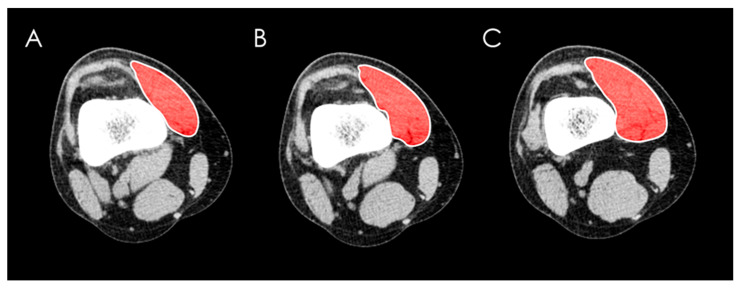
Measurement of the cross-sectional area of the vastus medialis obliquus (VMO) muscle by manually tracing its boundaries using the “area” tool on CT images at three levels: (A) the first ascending slice where the patella was no longer visible superiorly, (B) 1 cm above this slice, and (C) 2 cm above the first slice.
